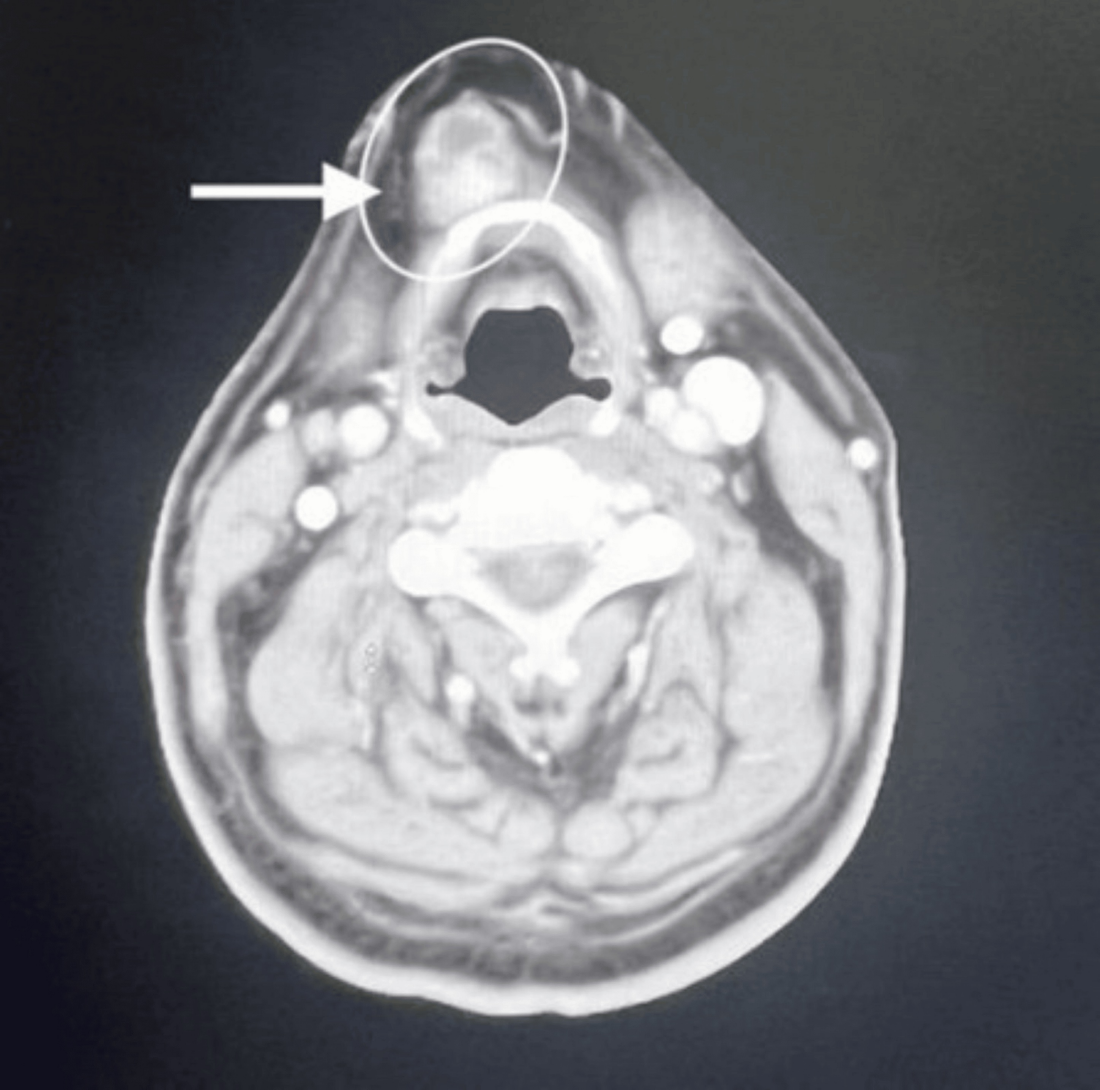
A contrast-enhanced axial CT scan at the hyoid bone level shows a multilocular cystic mass located in the midline of the neck, causing pressure on the airway. There is no evidence of regional lymphadenopathy, and the neck blood vessels are clear.
